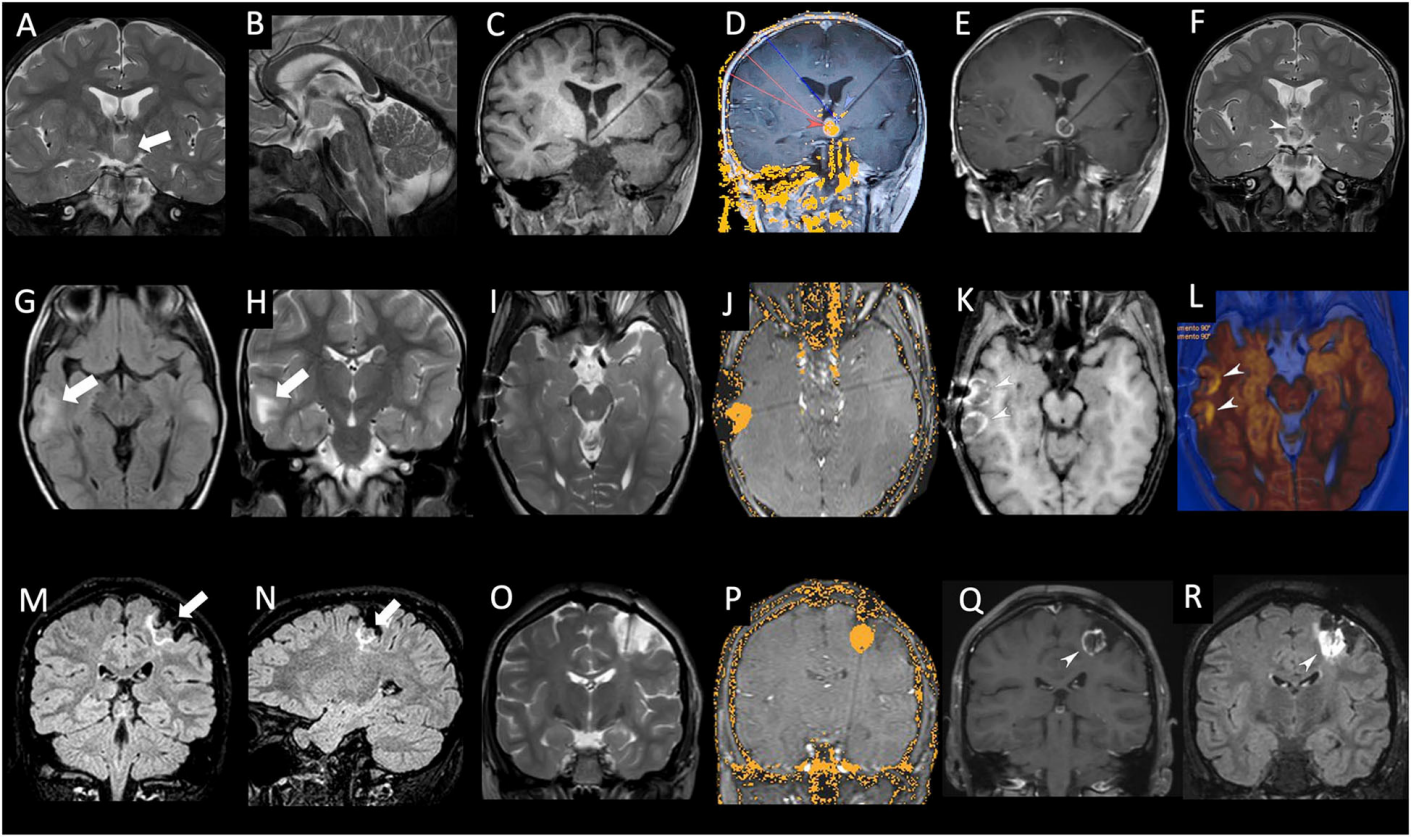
(A–F): Patient #2 (see Table 1), male, 2 years old. Coronal (A) and sagittal (B) T2-weighted images showing the intraventricular hypothalamic hamartoma localized on the left side (thick white arrow). Coronal T1-weighted image (C) acquired after stereotactic placement of a laser cannula within the hamartoma. Real-time MR thermogram overlaid on background T1-weighted image (D) exhibiting the irreversible damage map (yellow area within the hamartoma). Note the low-limit threshold, set at 48°C (blue arrowhead), placed on the left mammillothalamic tract, and the high limit-thresholds, set at 90°C (red arrowhead) at the tip of laser catheter and within the hamartoma. Coronal post-contrast T1-weighted image (E) performed at the end of laser ablation showing central necrosis of ablated hamartoma with peripheral contrast enhancement. Coronal T2-weighted image (F) performed 48 h after laser ablation confirming necrosis of ablated hamartoma (white arrowhead). (G–L): Patient #5 (see Table 1), male, 6 years old with tuberous sclerosis. Axial FLAIR (G) and coronal T2-weighted (H) image showing two cortical tubers in the right temporal lobe (white arrow). Axial T2-weighted image (I) acquired after stereotactic placement of two laser cannulas within the cortical tubers. Real-time MR thermogram overlaid on background T1-weighted image (J) exhibiting the irreversible damage map (yellow area within the tuber). Axial post-contrast T1-weighted image (K) performed at the end of laser ablation showing central necrosis of ablated tubers with peripheral contrast enhancement. Axial diffusion weighted image (L) overlaid on the axial T2-weighted image, performed at the end of laser ablation confirming necrosis of ablated tubers with peripheral restricted diffusion (white arrowheads). (M–R): Patient #6 (see Table 1), female, 15 years old. Coronal (M) and sagittal (N) FLAIR images showing relapsing DNET localized in the left post-central gyrus (thick white arrows). Coronal T2-weighted image (O) acquired after stereotactic placement of a laser cannula within the lesion. Real-time MR thermogram overlaid on the background T1-weighted image (P) exhibiting the irreversible damage map (yellow area within the hamartoma). Coronal post-contrast T1-weighted (Q) and FLAIR image (R) performed at the end of laser ablation showing central necrosis of ablated lesion with peripheral contrast enhancement (white arrowhead).
